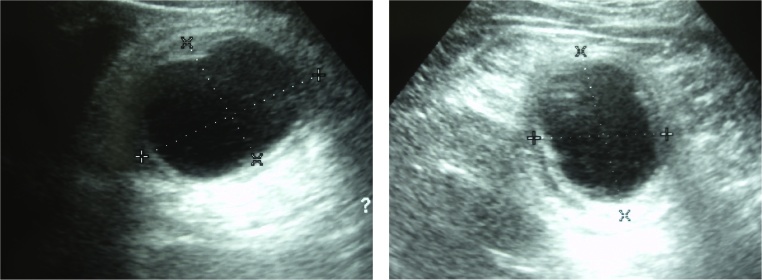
Abdominal ultrasonography showing an oval-shaped hypoechoic lesion in the right lower quadrant area.
